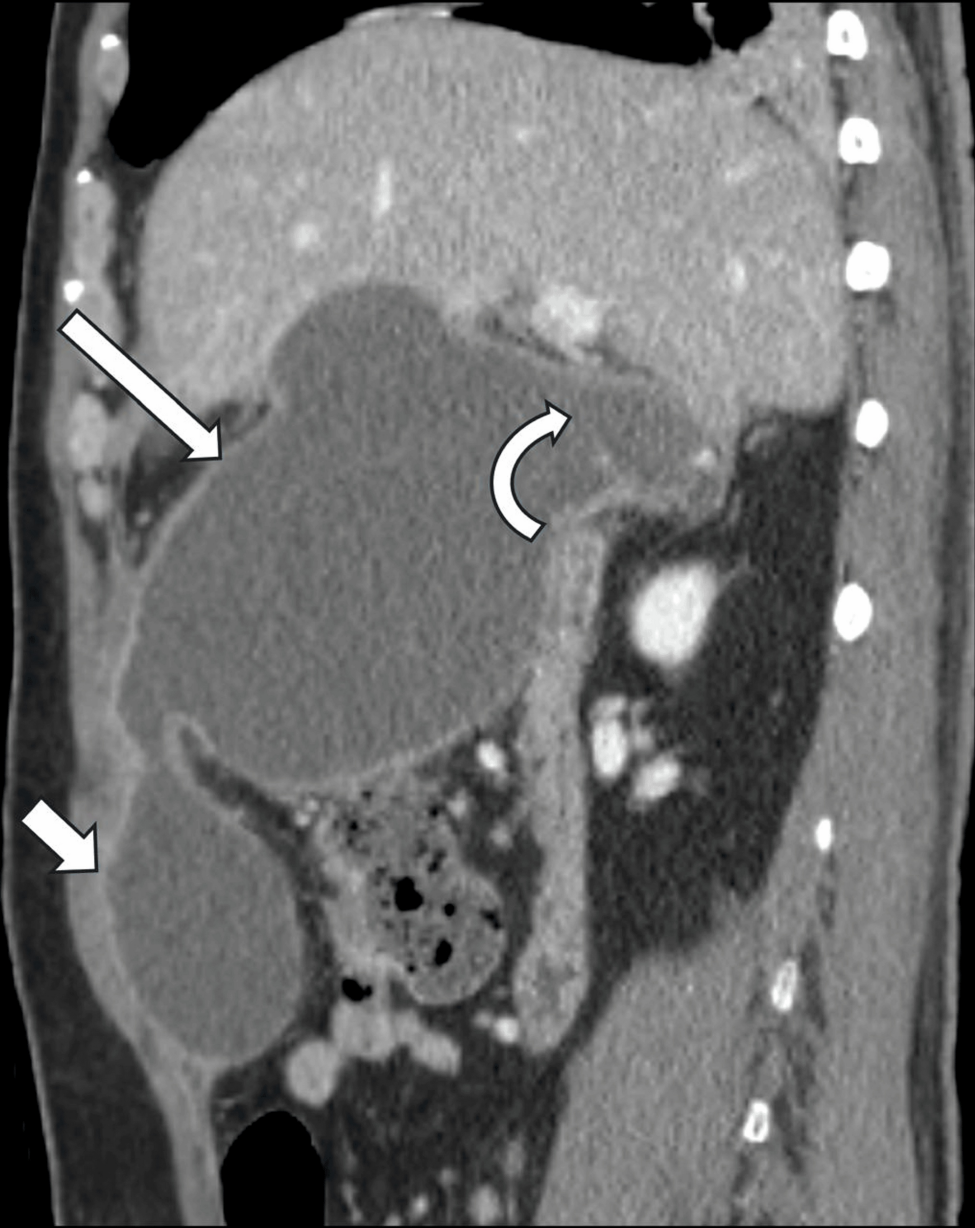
Follow-up contrast-enhanced abdominal CT scan (sagittal image) Follow-up contrast-enhanced abdominal CT scan (sagittal image) showing gallbladder wall defect (curved arrow) associated with two large interconnected rim-enhancing extrahepatic fluid collections (long and short arrows). The larger fluid collection measures 9.8 x 13.5 x 15.7 cm. The appearance is consistent with perforated cholecystitis with extrahepatic infected bilomas/abscesses.
